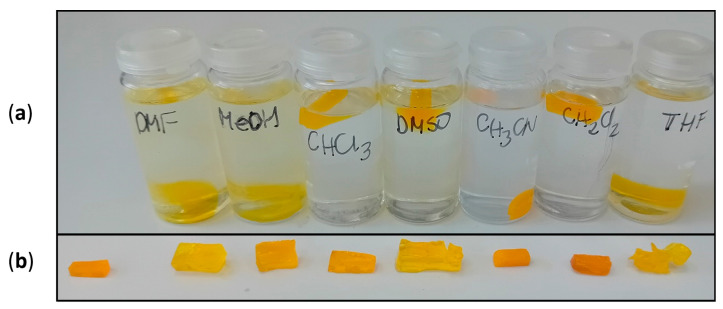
(a) The appearance of IL-NIPU1 in solvents after exposure to the following conditions: an ultrasound at a temperature of 50 °C for 10 h; and (b) the appearance of IL-NIPU1 after removing it from the solvents. On the left is a reference piece of NIPU.
